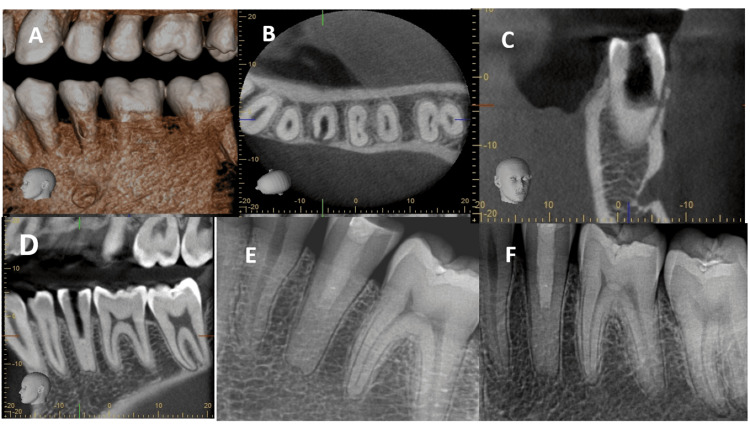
Case 2 - cone-beam computed tomography of tooth #20 (A) Cone-beam computed tomography (CBCT) of tooth #20 (October 2020). (B) Axial view of the same CBCT showing buccal root perforation of tooth #20. (C) Sagittal view of the same CBCT showing buccal root perforation of tooth #20. (D) Coronal view of the same CBCT of tooth #20. (E) Radiograph post-perforation repair using bioceramic material. (F) Recall radiograph (March 2021) showing healing of periapical tissues and no signs of disease progression.
